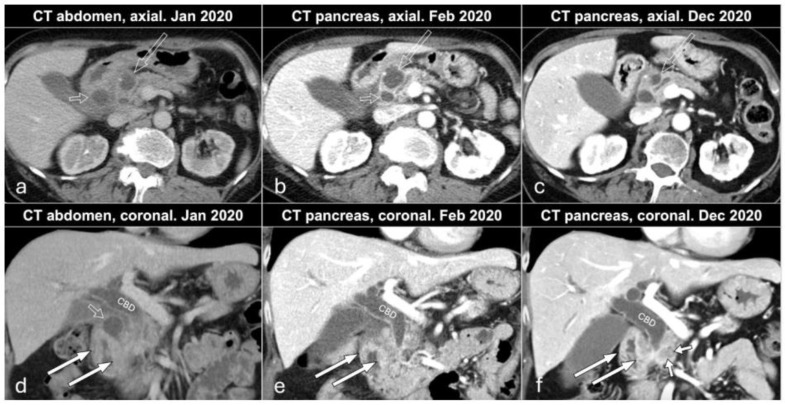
Axial (a–c) and coronal (d–f) contrast-enhanced images of CT abdomen in January (a,d) and CT pancreas in February (b,e) and December (c,f) 2020. CBD: common bile duct; open white arrows: cystic lesion in the pancreatic head; open short white arrows: cystic lesion in the pancreatic groove; white arrows: wall thickening of the descending portion of duodenum; short white arrows: pancreatic cancer.
